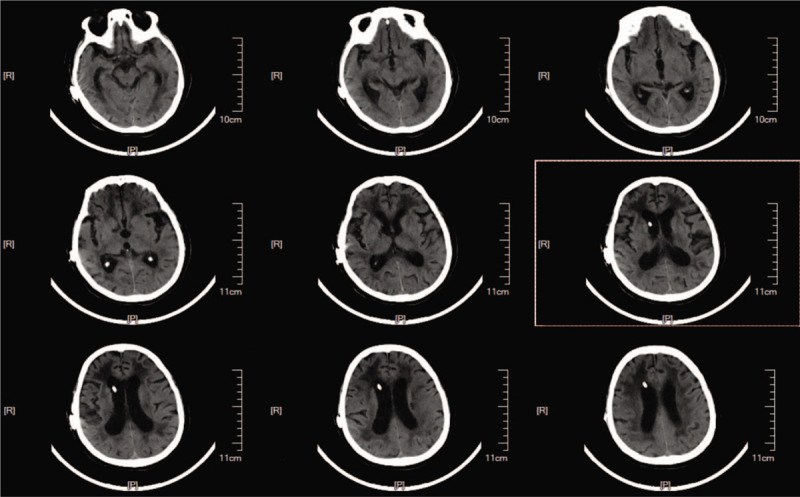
Preoperative head computed tomography scan obtained during the second admission indicated ventricular enlargement and the recurrence of obvious paraventricular edema.
